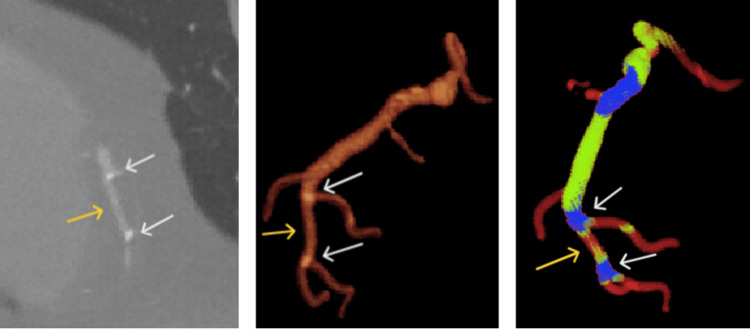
Right coronary artery with mixed etiology plaque; the white arrows indicate calcified components, whereas the yellow arrows indicate the soft components of mixed plaques.  (left) Oblique CT slice of the plaque; the white arrows point to the calcified regions (high attenuation), and the yellow arrows point to the approximate location of the softer (lipid/fibrotic) components (lower attenuation). (middle)  The 3D volume rendering displays the geometry of the artery and the extent of the plaque. (right)  Image of the clustering analysis results; calcified regions appear in a blue cluster, while the softer plaque portions blend with narrower vessel segments (orange/red clusters).
